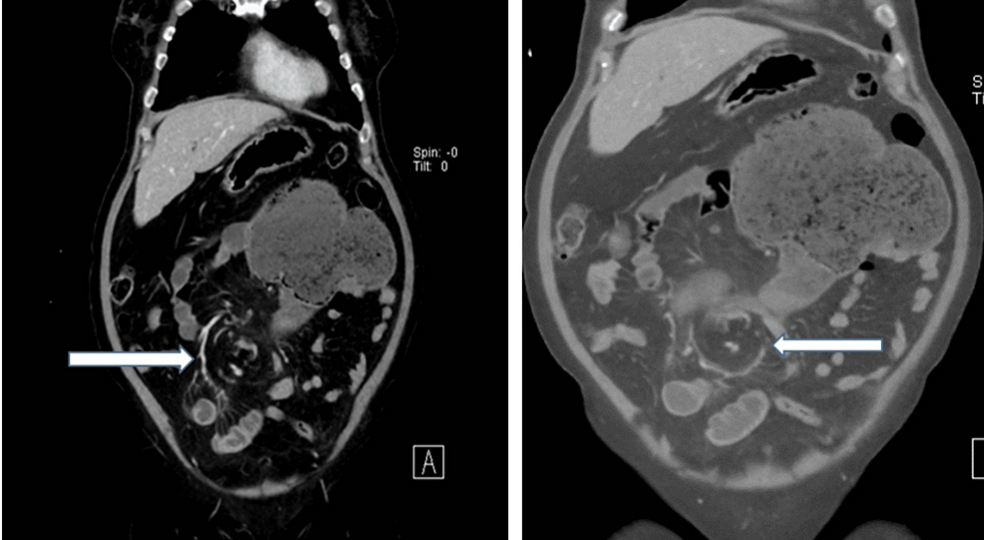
Two (coronal view) of the CT abdomen showing the whirl Sign (white arrows).
